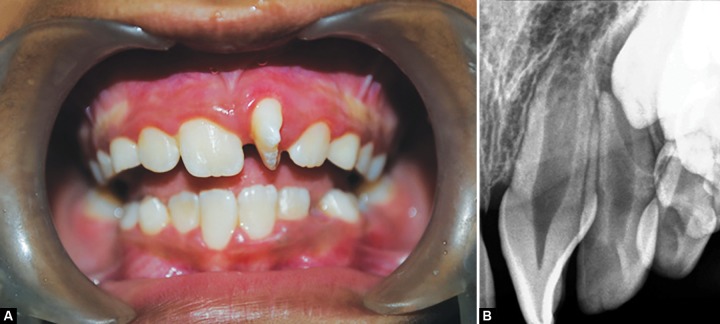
(A) Clinical picture depicting maxillary left central incisor (21) rotated mesiopalatally and (B) intraoral periapical digital radiograph showing absence of any supernumerary tooth and incomplete development of root of both 11 and 21
